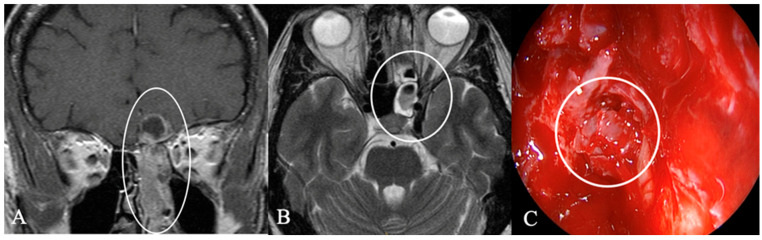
Olfactory neuroblastoma. A 57-year-old female presented with nasal breathing obstruction. (A) Coronal contrast-enhanced MRI and (B) T2 MRI reveal a heterogeneous, partly cystic tumorous lesion involving the sellar region and paranasal sinuses. (C) Intraoperative image demonstrating infiltrative growth. Postoperatively, radiotherapy (up to 54 Gy) was administered. Seventy-two months later, submandibular metastases and a diffuse cerebral, dural spread were observed.
